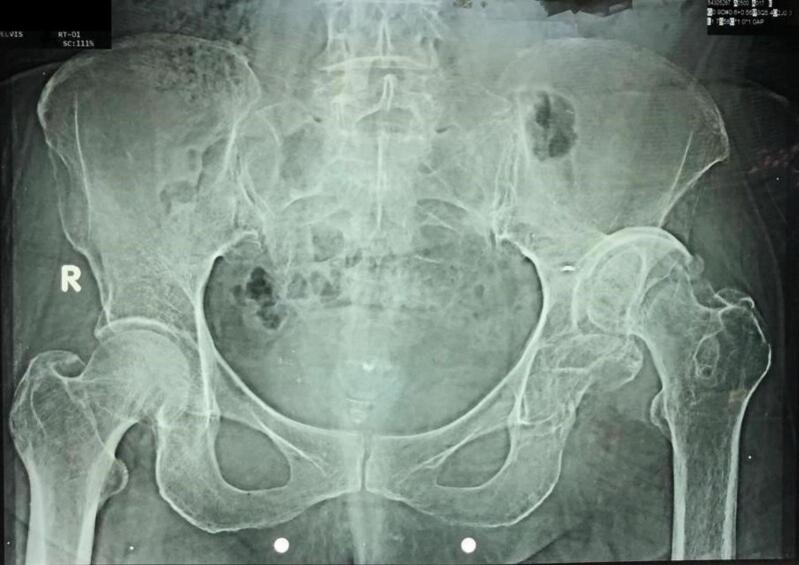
A plain radiograph shows a neglected left posterior hip dislocation with a false acetabulum and a fracture of the acetabular floor.
